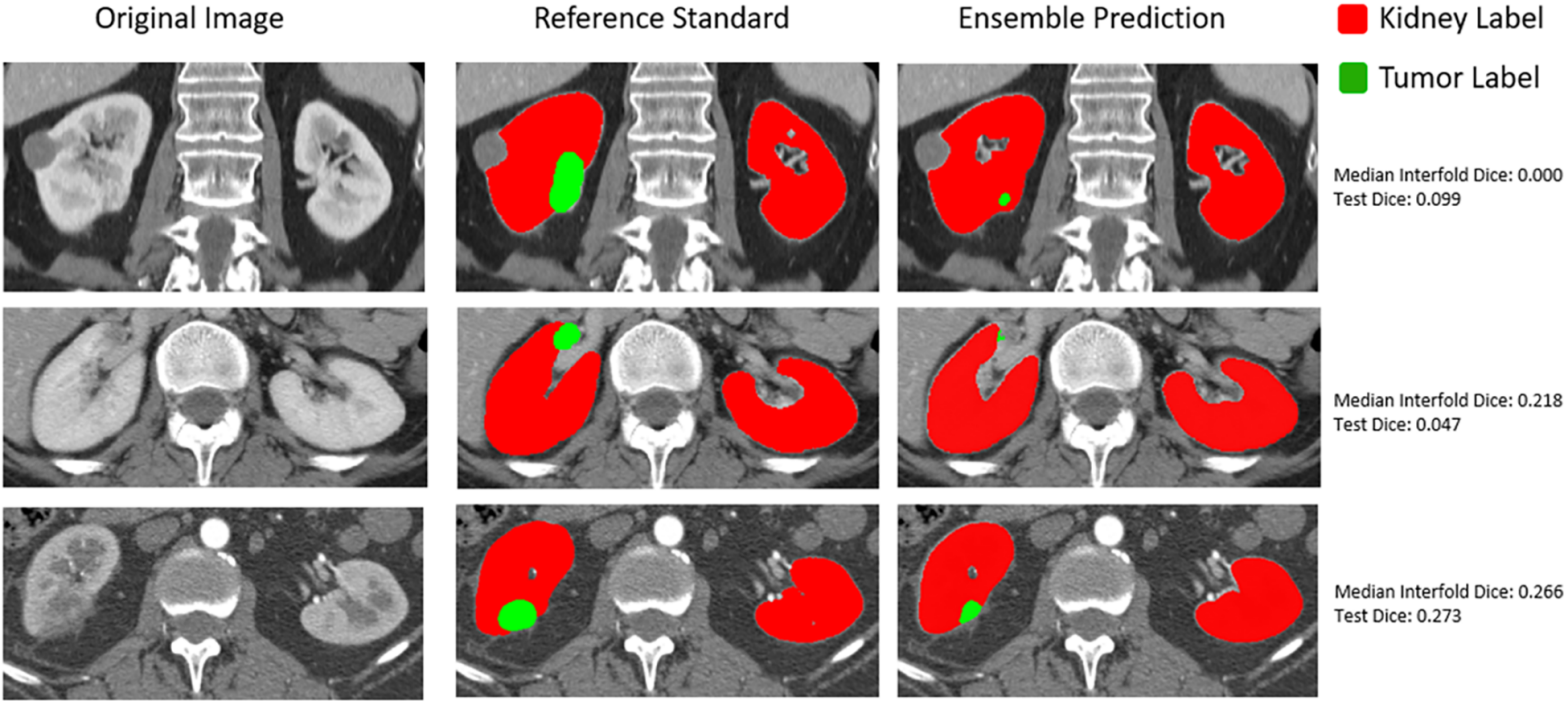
Qualitative assessment of outliers in internal CT tumor test set shown in the lowest left quadrant of Figure 4.
